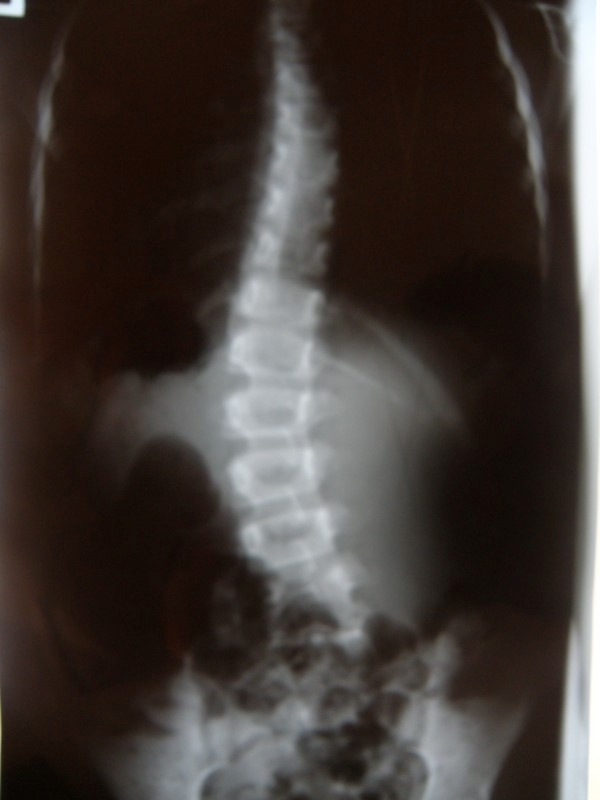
Radiography before treatment.
